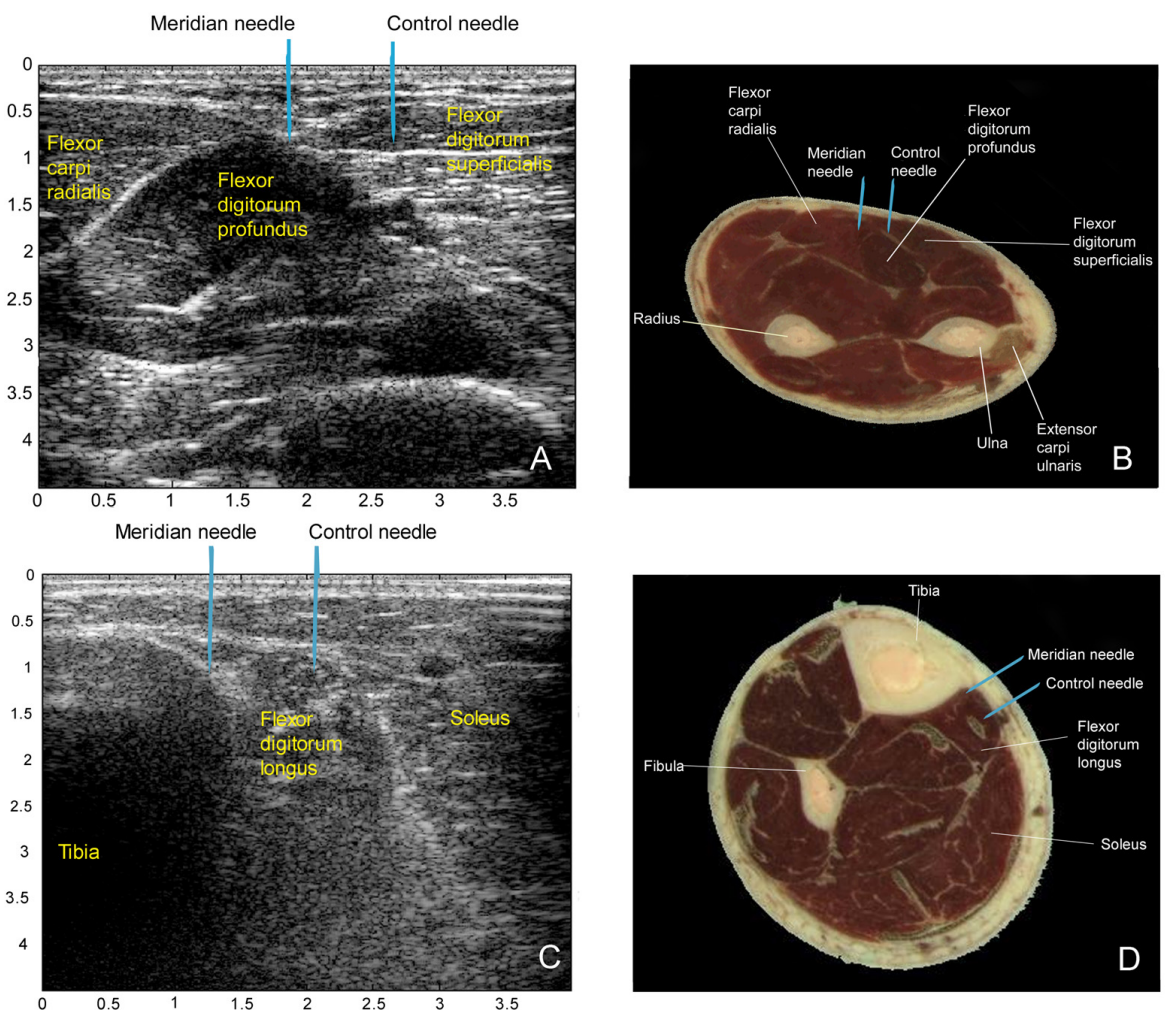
Depiction of the Spleen and Pericardium meridians in relation to surface and sub-surface anatomical landmarks. 2 A, B: Cross sectional images of the right forearm as shown through ultrasound and gross anatomical cross section (obtained from the Visual Human Database). The Pericardium segment is located between the flexor carpi radialis and flexor digitorum superficialis muscles. 2 C, D: Cross sectional images of the right leg as shown through ultrasound and cadaveric cross section. The Spleen segment is located between the medial crest of the tibia and the flexor digitorum longus muscle. Blue arrows point to approximate sites where meridian and control needles were inserted.
